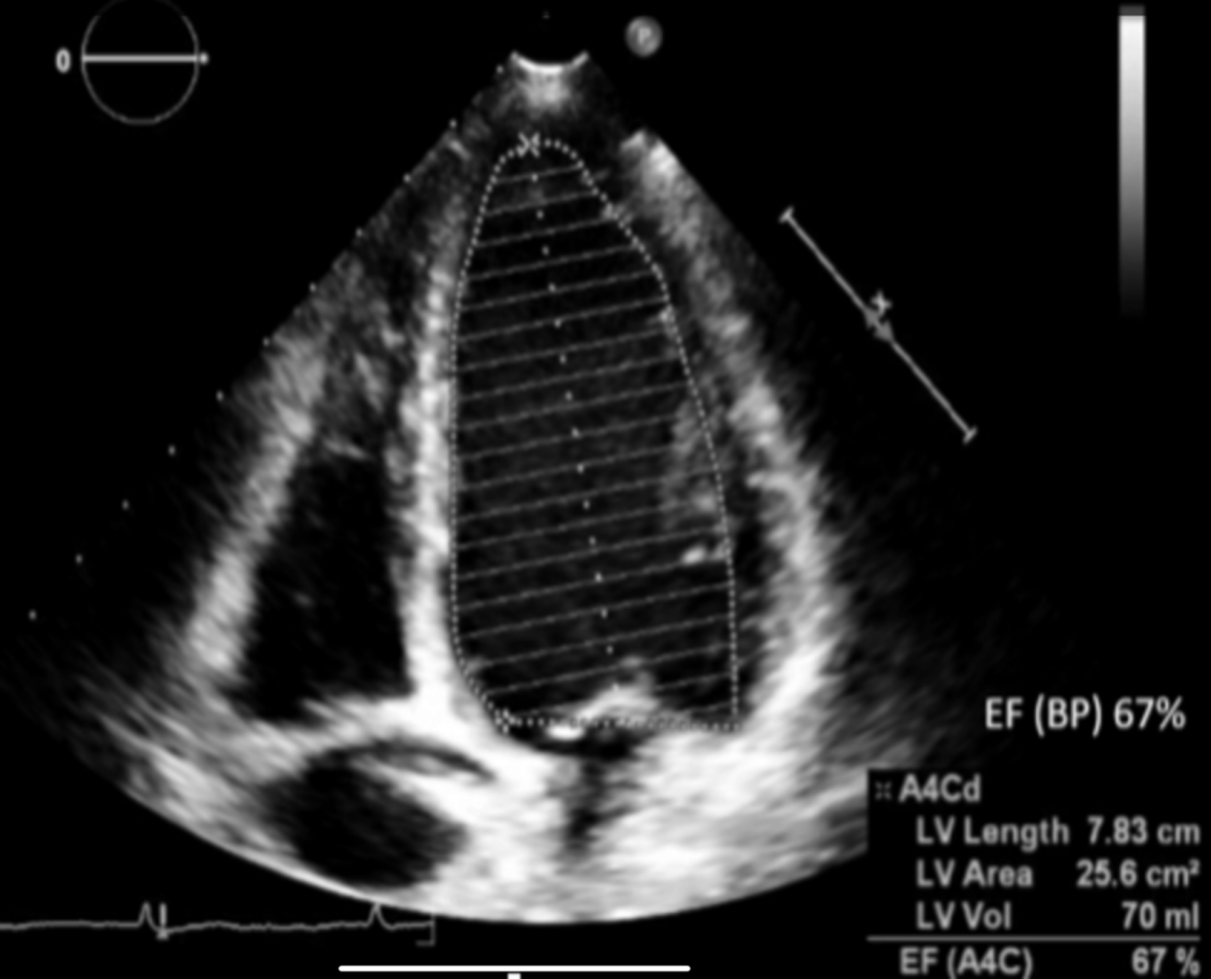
Transthoracic echocardiogram: apical 4-chamber view, LVEF of 67% as calculated by Biplane Simpson's method BP: biplane Simpson's method, A4Cd: apical four-chamber diastolic view, LV: left ventricle, EF: ejection fraction
